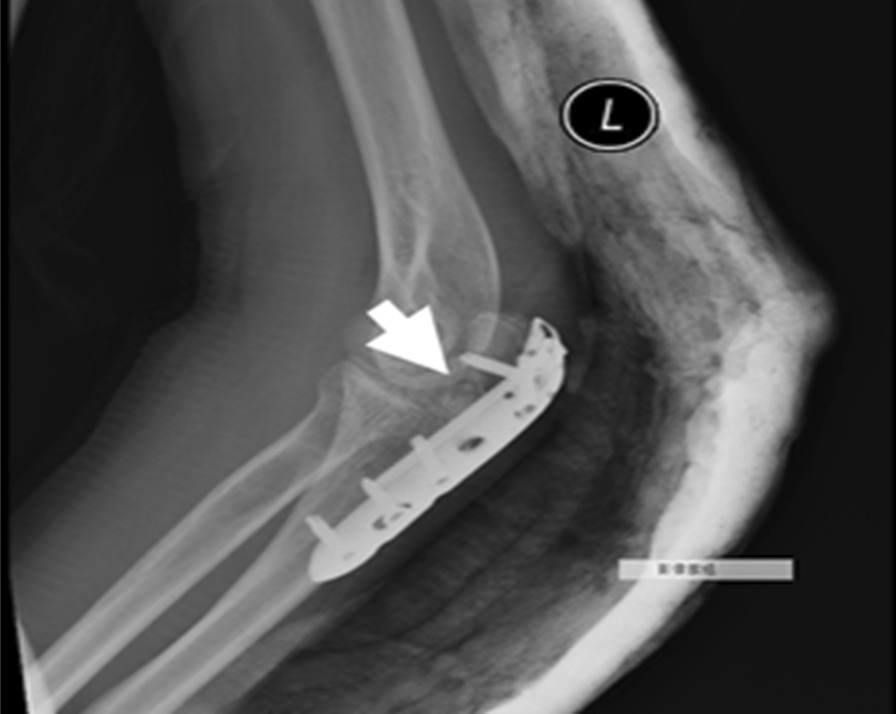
The nidus-like appearance (white arrow) in the X-ray indicates a primary fusion of the bone substitute and its close contact with the surrounding bone
